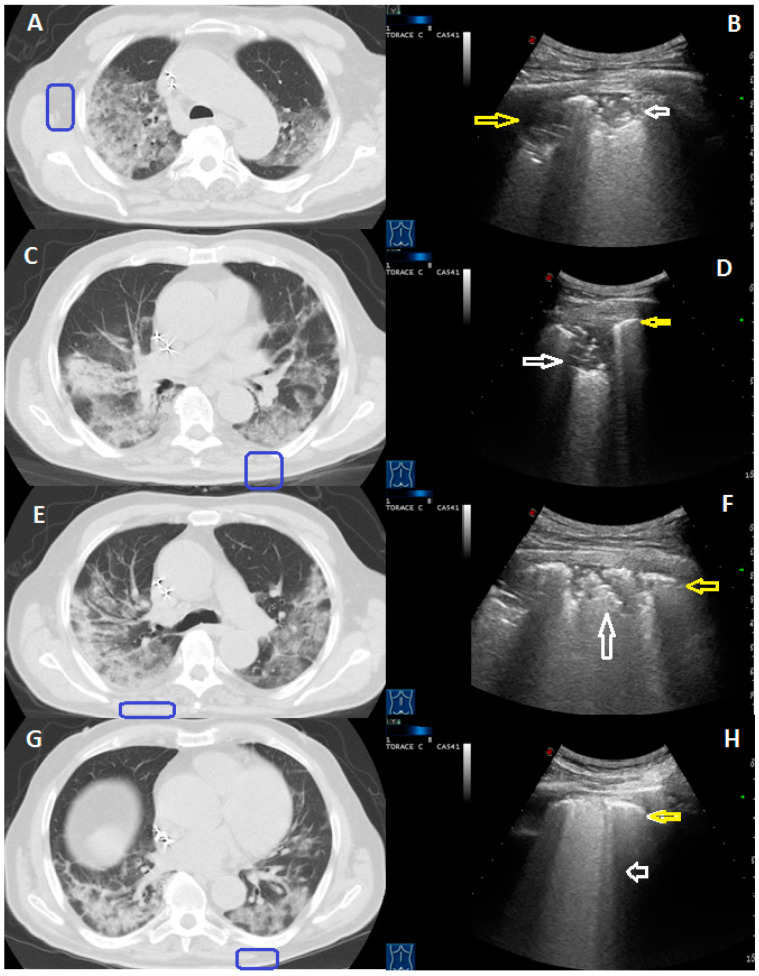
A 78-year-old male patient presenting with fever and cough for 10 days. The RT-PCR assay confirmed the suspect for COVID-19 pneumonia. The peripheral subpleural consolidation showed in (A) CT scan was in part located in the retroscapular area (blue box). The corresponding ultrasound scan with a convex probe (6 MHz) and thoracic setting in (B) allows us to view its non-retroscapular part as a mixed hyper-hypoechoic subpleural area (white arrows). Not all of the consolidations shown in (C,E,G) CT scans were adherent to the pleural surface (blue boxes). The corresponding ultrasound scans with a convex probe (6 MHz) and thoracic setting in (D,F) show a thickened hyperechoic pleural line (yellow arrow), with a mixed hypo-echoic subpleural consolidation (white arrow), that represent the adherent to the pleural surface part of these consolidations. On the contrary, ultrasound scan in (H) allows us to view only a blurred and thickened hyperechoic pleural line (yellow arrow), with B line below it (white arrow).
